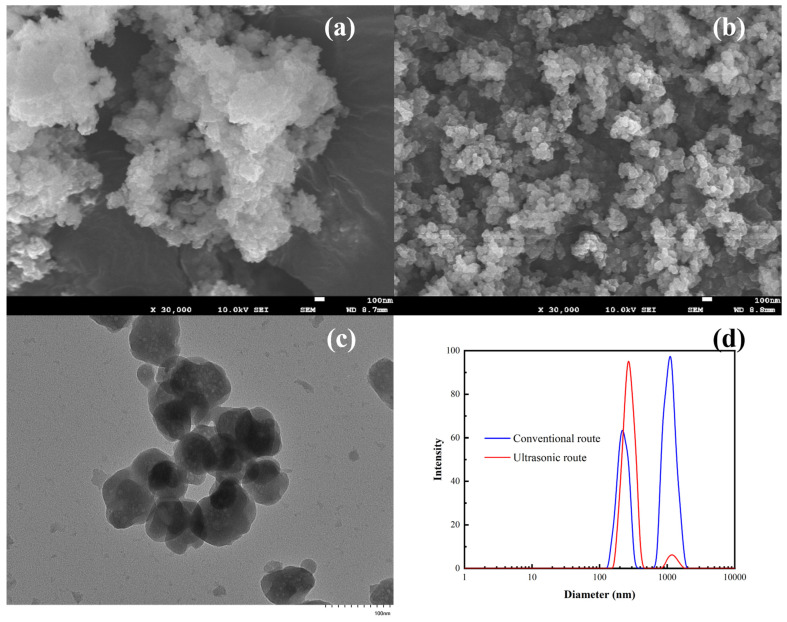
SEM images of iron phosphate precipitated via (a) conventional and (b) ultrasonic routes. (c) TEM image of the iron phosphate sample obtained via ultrasonic-assisted precipitation. (d) Particle size distribution of iron phosphate samples obtained using conventional and ultrasonic routes.
